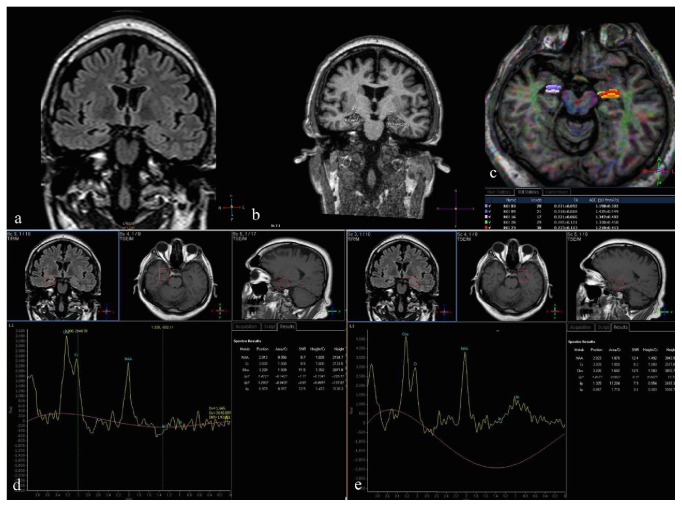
Quantitative and visual analysis of a right-sided TLE patient. Coronal FLAIR thin cut (2 mm) orthogonal to hippocampus. Right hippocampal gyrus is atrophic and shows increased intensity compared to left (a). 3D T1W coronal image shows reduced volume subjectively interpreted as atrophy. The coronal volumetric image shows reduced area on the right hippocampus as well (b). DTI and 3D T1W axial fused image shows reduced FA and increased ADC values at right hippocampus compared to left (c). Right hippocampal gyrus (d) shows reduced Cho/Cr and NAA/Cr compared to left (e) (please note that lactate is absent).
